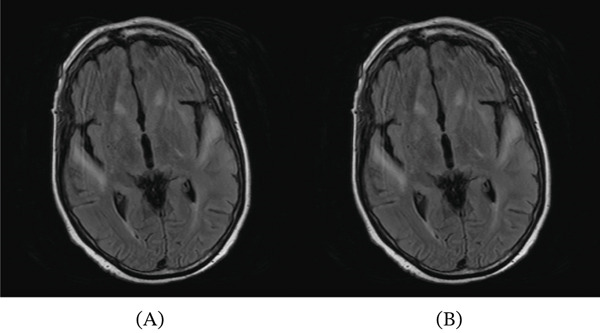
(A, B) Hospital day 14 MRI T2/FLAIR sequences redemonstrating previously visualized enhancement.
